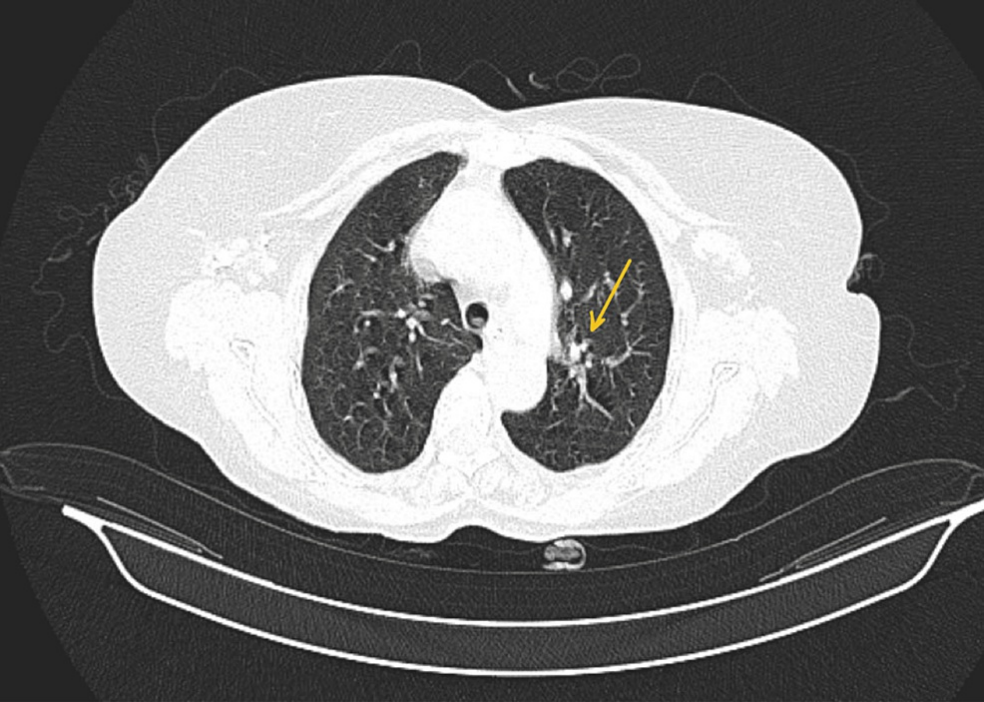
Pre-treatment HRCT showing ground glass opacities in the posterior basal segment of the left lower lobe, subsegmental atelectasis in the inferior lingular segment of the left upper lobe (yellow arrow), and fibrotic changes in the left lower lobe HRCT: High-resolution computed tomography
